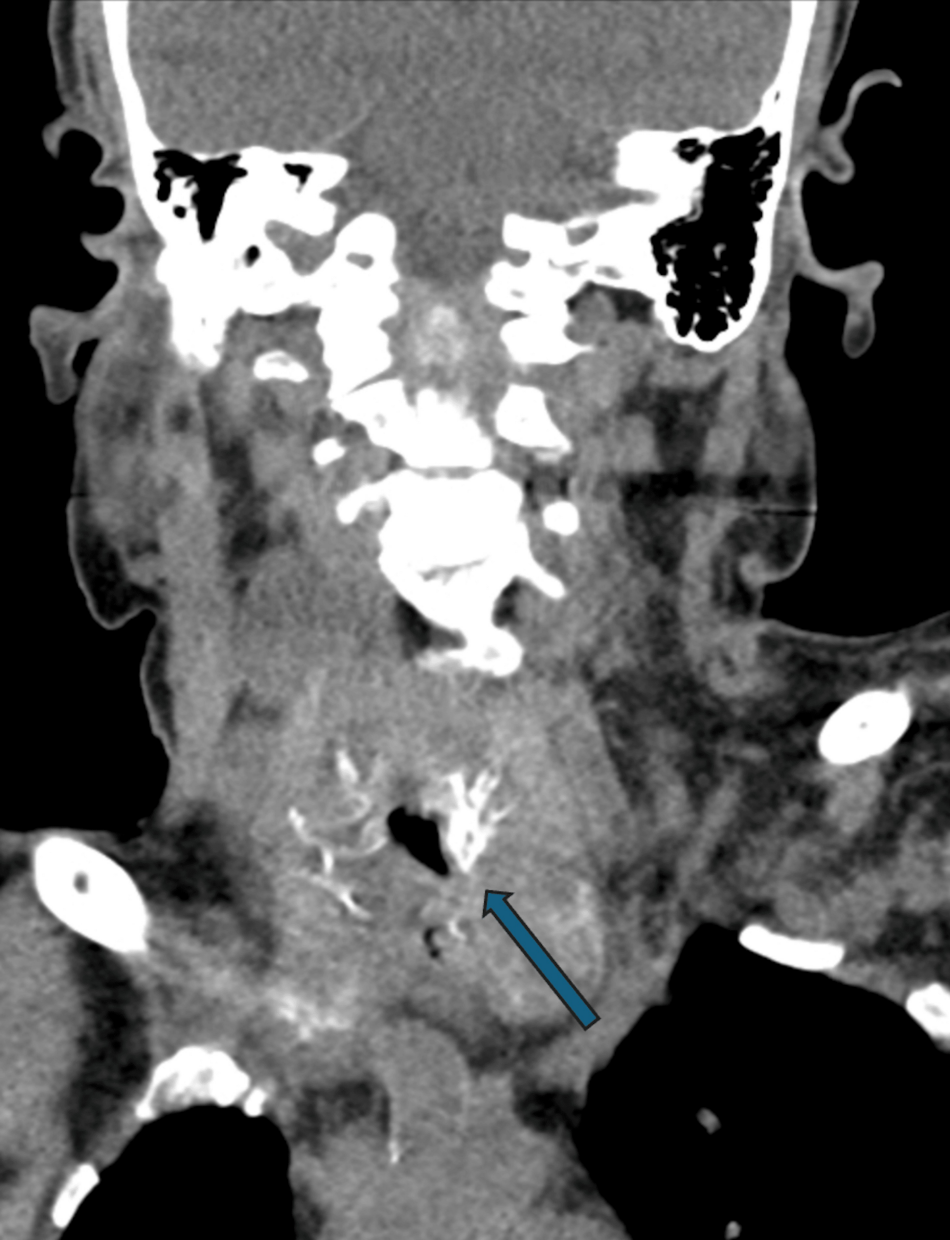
Coronal CT of the neck demonstrating a tracheostomy defect in the lower anterior mid-neck. Subglottic airway narrowing is present with associated soft tissue density surrounding the airway (blue arrow).
